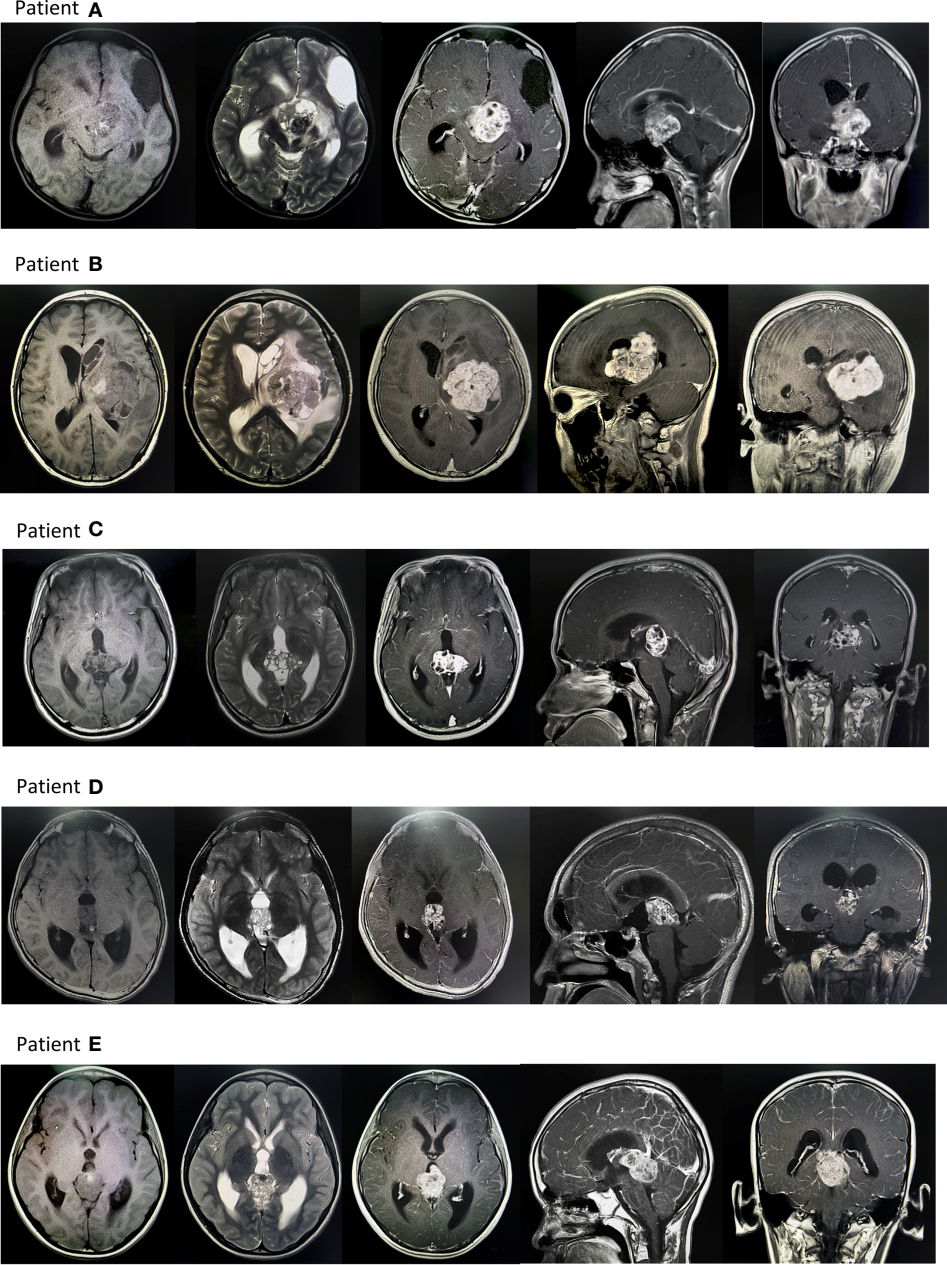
Typical MR images of intracranial germ cell tumors patients. Patient (A) There was an irregular cystic solid tumor in the suprasellar area, with a size of about 40mmx35mmx34mm, with clear borders, high and low mixed signals on T1WI, and high and low mixed signals on T2WI. After enhanced enhancement, the possibility of a germ cell tumor was considered. There are fusiform nodules in the left ventricle and the fourth ventricle, with clear borders, about 12mmx8mmx7mm, 9mmx8mmx16mm in size, and the enhancement is obviously uneven, and implantation is considered. Patient (B) Huge mass in the left basal ganglia with poorly defined borders, the largest slice is about 53mmx69mm: TIWI with uneven low signal, see strip-like high signal, T2WI with uneven high signal, see patch cystic lesions, uneven after enhanced scan Significant enhancement. Edema zone was seen in the brain parenchyma around the tumor. The possibility of germ cell tumor was considered. The left lateral ventricle was compressed and narrowed, and the midline structure was shifted to the right. Patient (C) An irregular mass was seen in the pineal region, with a size of about 24mmx35mmx32mm, with a clear boundary and uneven signal. T1WI showed equal and slightly low signal, and T2WI showed slightly high signal. The solid component was significantly enhanced on enhanced scan. The third ventricle is dilated and effusion, thus it is likely to be a reproductive tumor. Patient (D) Irregular soft tissue signal mass can be seen in the pineal region, with a clear boundary and a range of about 21mmx27mmx20mm. T1WI shows a slightly low signal, a few dots and a slightly high signal inside, T2WI shows a slightly high signal, and it shows uneven enhancement after enhancement, and germ cell tumor is considered. tumor. Obstructive hydrocephalus of the third ventricle and bilateral lateral ventricles. Patient (E) An irregular mass foci was seen in the pineal region, about 32mmx30mmx36mm in size. TIWI showed a low signal, and T2WI showed a mixed abnormal signal area of equal height, in which the liquid level was seen, the enhancement was obvious, and the cystic area was seen, and a germ cell tumor was considered. Occupying the great cerebral venous cistern, pushing the quadrigeminal cistern, and protruding into the third ventricle, compressing the midbrain aqueduct, causing double ventricles, dilation of the third ventricle, and obstructive hydrocephalus.
